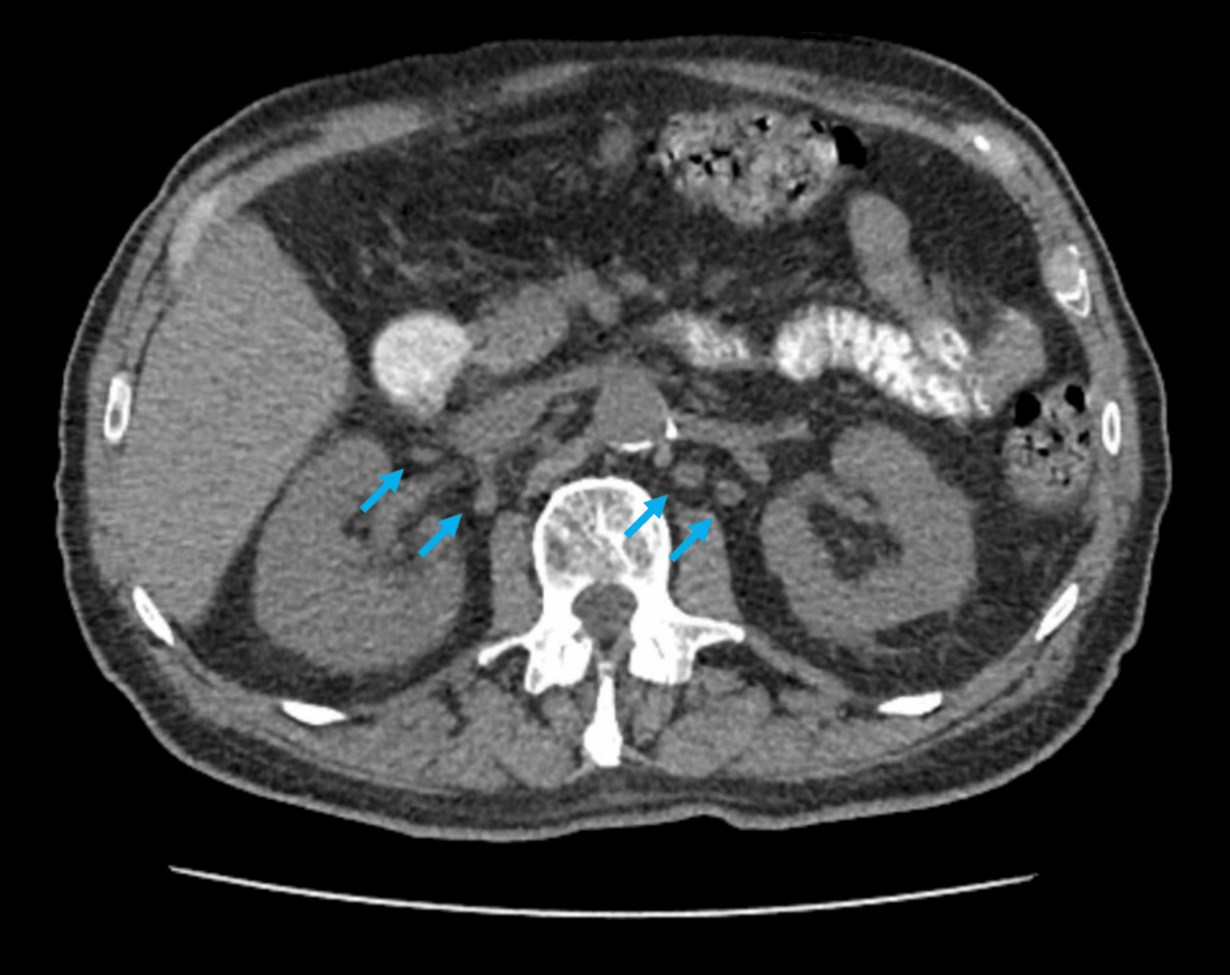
Abdominal CT CT scan showing multiple abdominal lymphadenopathies (arrows).
